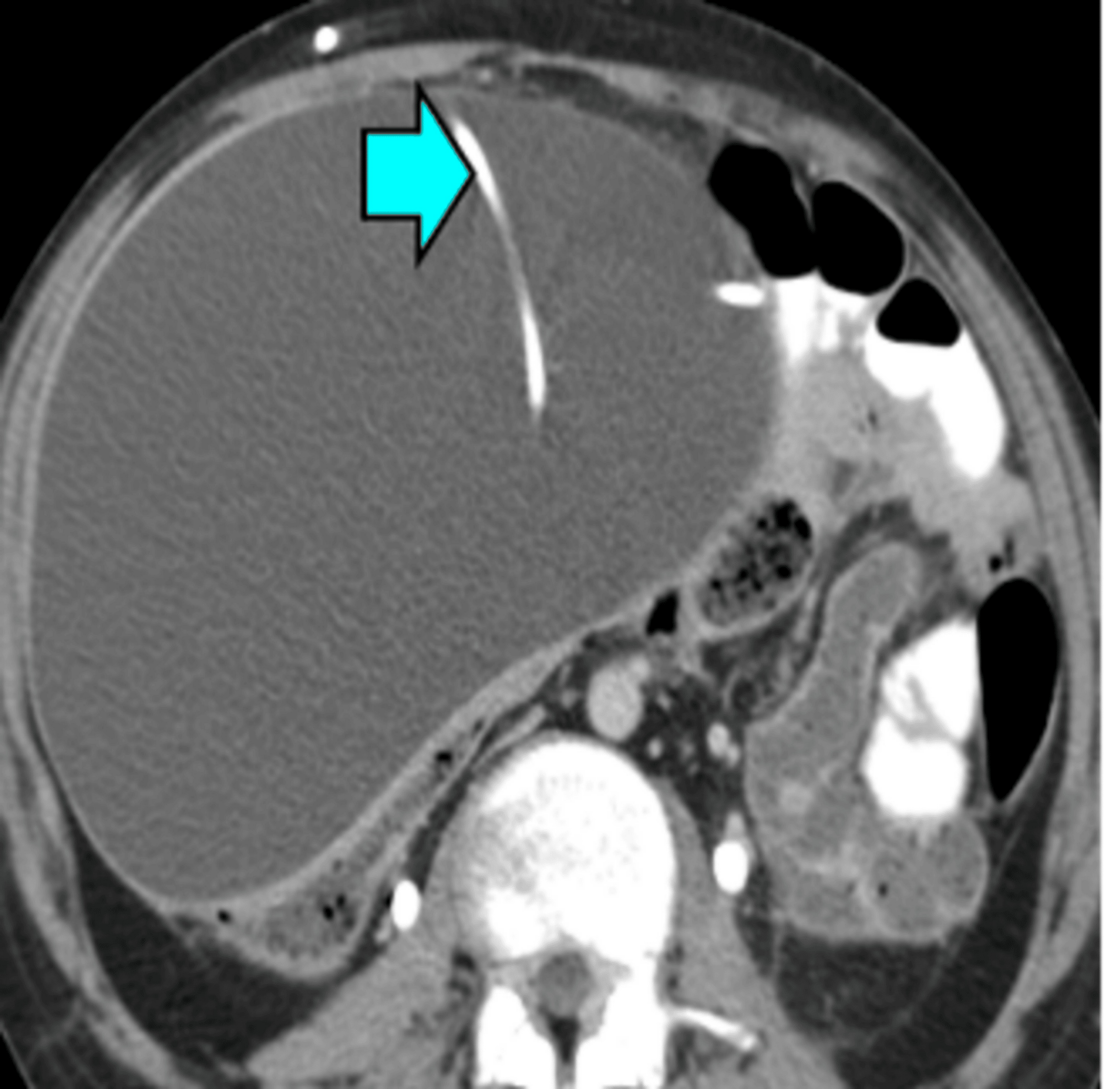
CSF pseudocyst. Large fluid collection associated with a shunt (blue arrow) in the peritoneal cavity on CT imaging.
